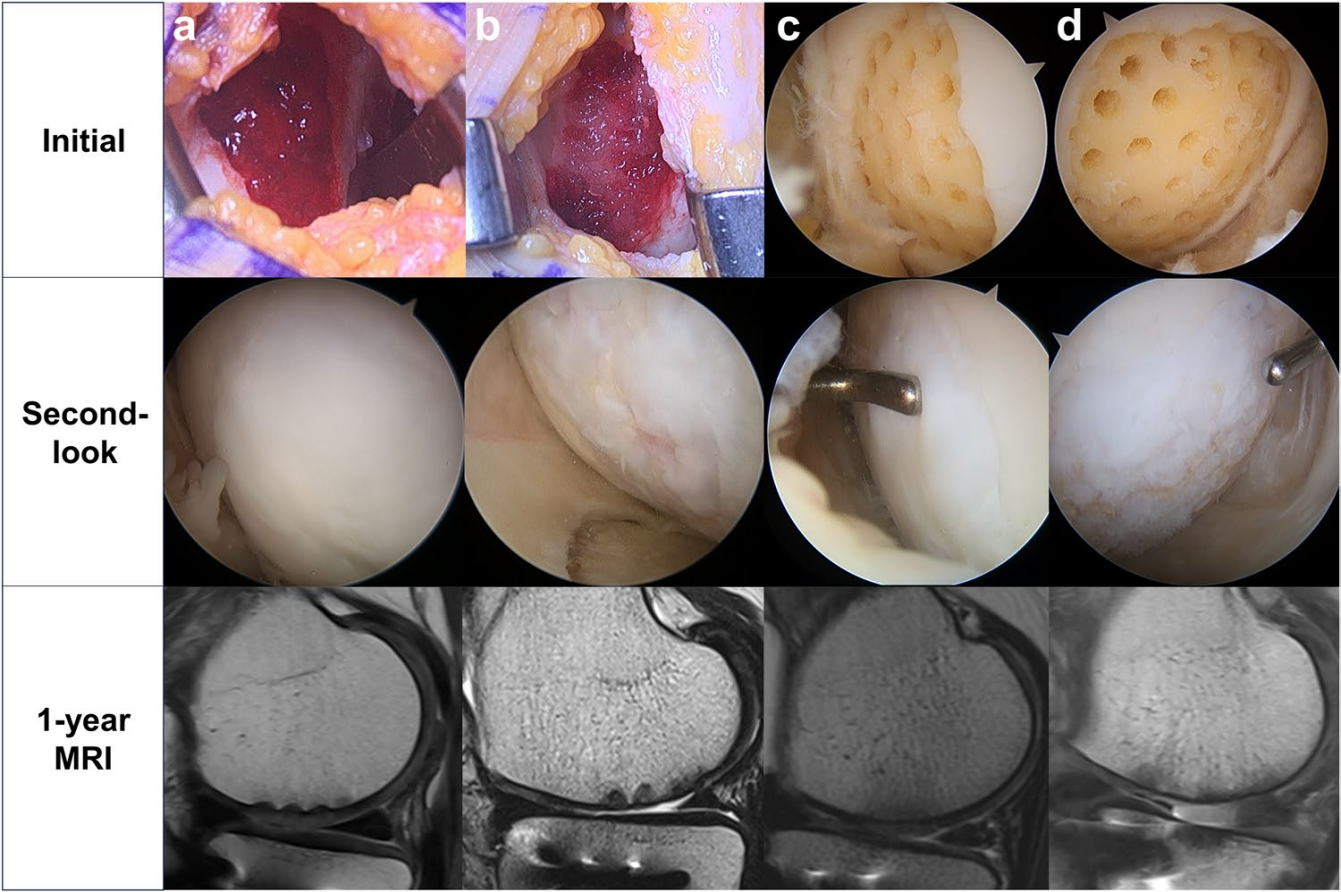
Cartilage regeneration outcomes in patients underwent two different procedures, hUCB-MSC implantation (a, b) and arthroscopic microdrilling (c, d). Initial, second-look arthroscopy, and postoperative MRI images depict the best (a, c) and the worst (b, d) cases among patients with anterior-to-mid lesions. (a) Fully covered medial femoral condyle (MFC) defect with cartilaginous tissue, achieving a MOCART score of 70. (b) Incomplete coverage of the MFC defect with irregular cobblestone appearance, resulting in a MOCART score of 35. (c) Complete coverage of the MFC defect after arthroscopic microdrilling, with softness on probing noted. (d) Minimal coverage of the MFC defect, presenting as soft and thin tissue, with a MOCART score of 25. MOCART, Magnetic Resonance Observation of Cartilage Repair Tissue.
